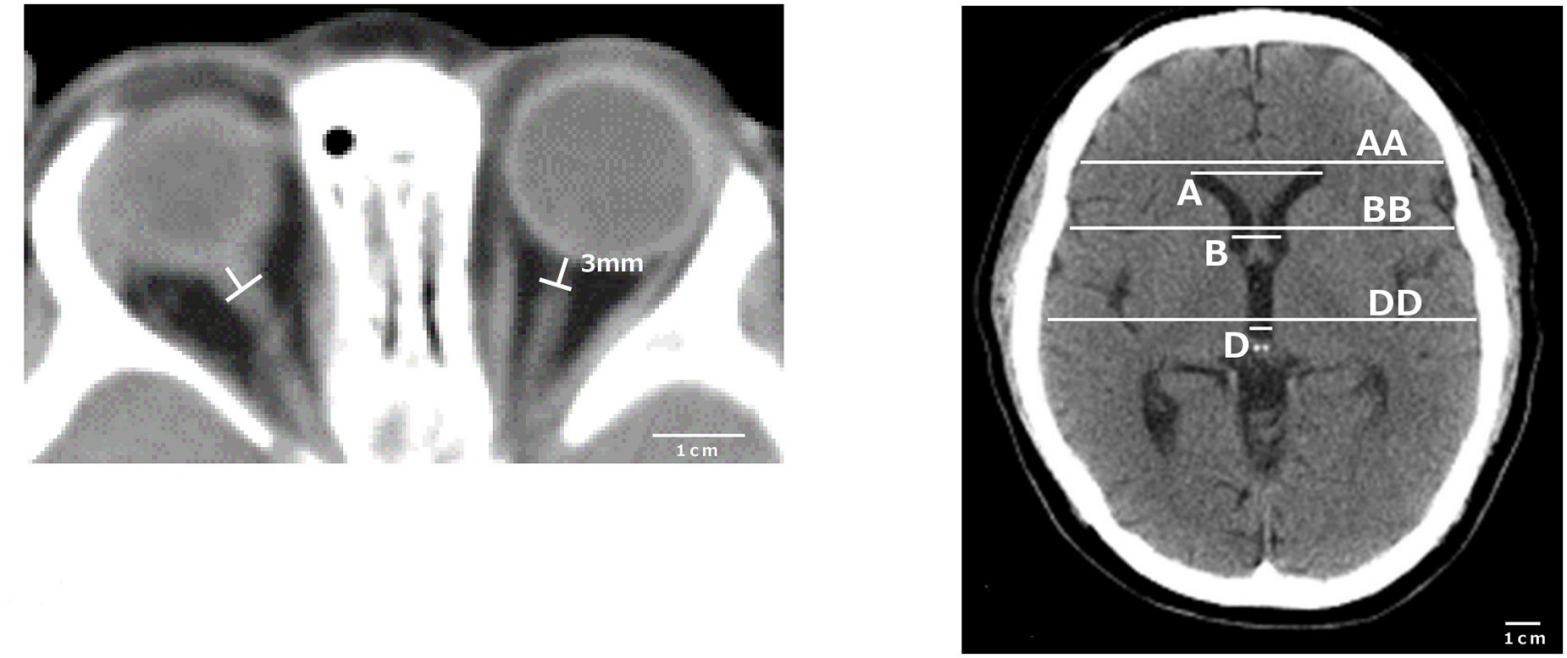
Computed Tomography imaging. The optic nerve sheath diameter was measured 3 mm behind the posterior aspect of the globe on axial sequences. A: Maximum distance between anterior horn, B: Minimum bicaudate nuclei distance, D: Third ventricle distance. AA, BB and DD were corresponding brain width, respectively. EE defined a square root of summation for AA2, BB2, and DD2.
